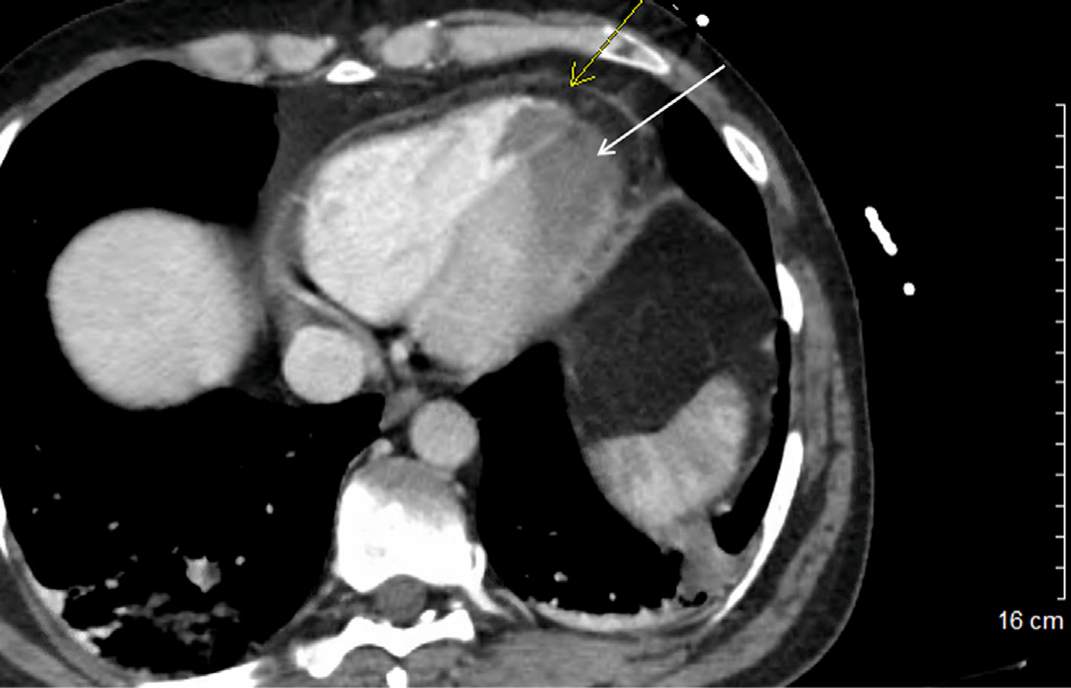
Biventricular Apical Thromboses Computed tomography scan showing left (white arrow) and right (yellow arrow) ventricular apical thromboses, a new finding compared with a computed tomography scan performed 2 months earlier.
